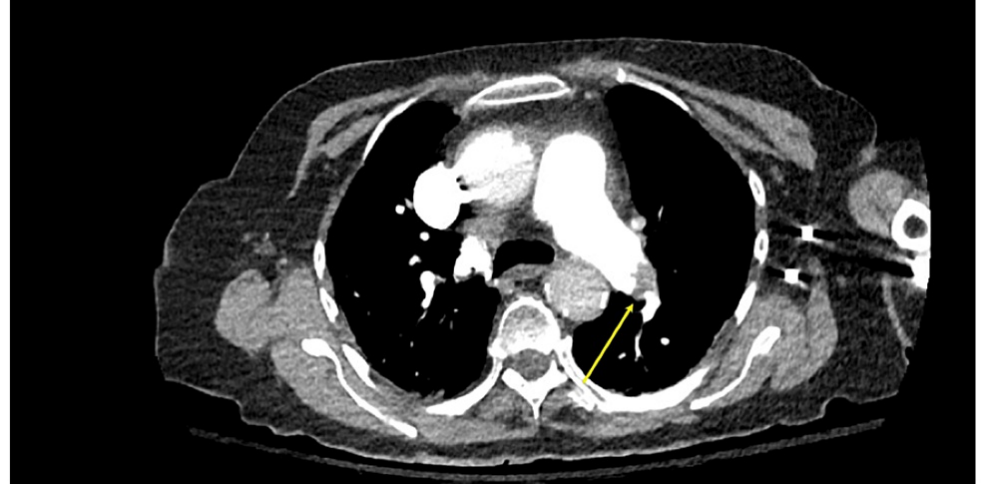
CT angiogram showing thrombus in the left pulmonary artery. Thrombus is seen here in the left pulmonary artery, confirming the diagnosis of a pulmonary embolus.
